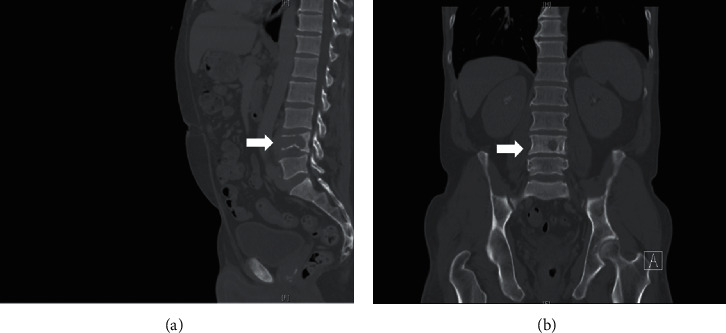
Lumbar CT, left sagittal (a) and coronal (b) planes. A destructive lesion causing pathological fracture of the L4 vertebral body with mild adjacent soft tissue thickening. No abnormal soft tissue in the spinal canal was identified.
